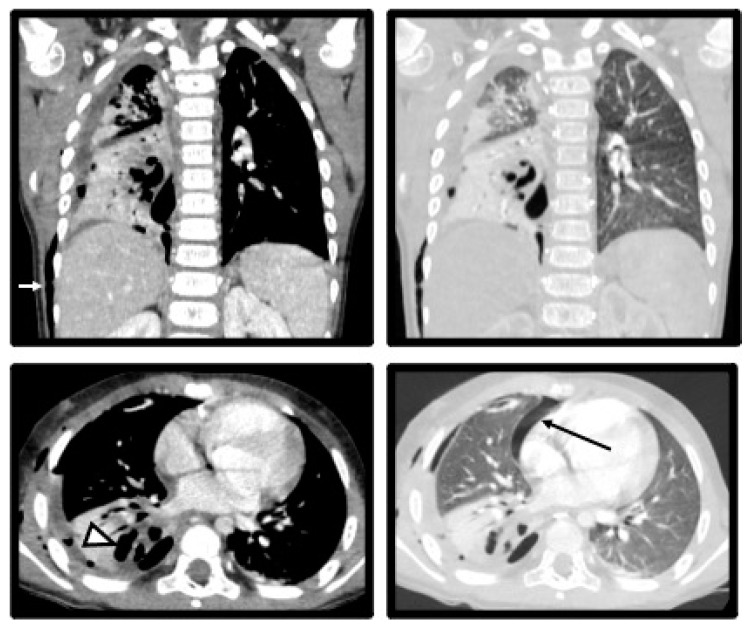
Day 7 of hospital admission. Chest CT (coronal and axial MPRs, mediastinal and lung windows) post-thoracocentesis reveals small residual pneumothorax (white arrow), pleural empyema with well-defined area of non-enhancing lung with cavitation adjacent to empyema (arrowhead) and subcutaneous emphysema (black arrow).
